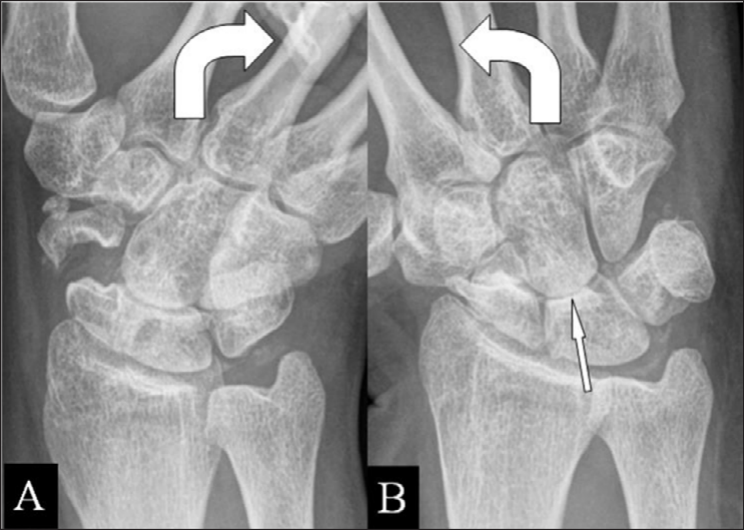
(Intra)scaphoid abutment. (A, B) PA plain mobility radiographs in ulnar and radial deviation. (A) Large diastasis in ulnar deviation between the scaphoid bone fragments. (B) Impaction of both fragments in radial deviation. Associated midcarpal osteoarthritis (arrow).
